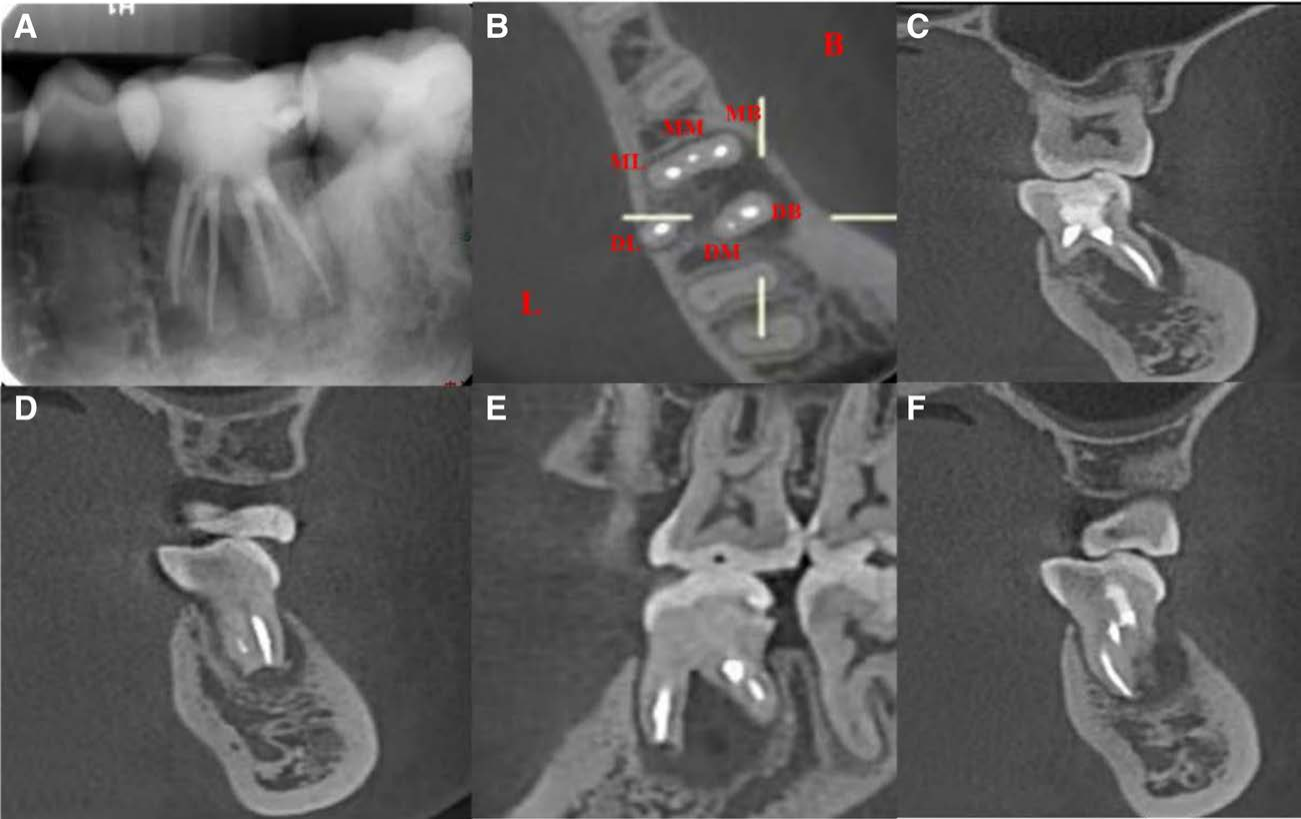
The immediate postoperative periapical radiographs and CBCT images of tooth 36. (A) Postoperative periapical radiographs. (B–F) Postoperative CBCT images. CBCT = cone-beam computed tomography.
